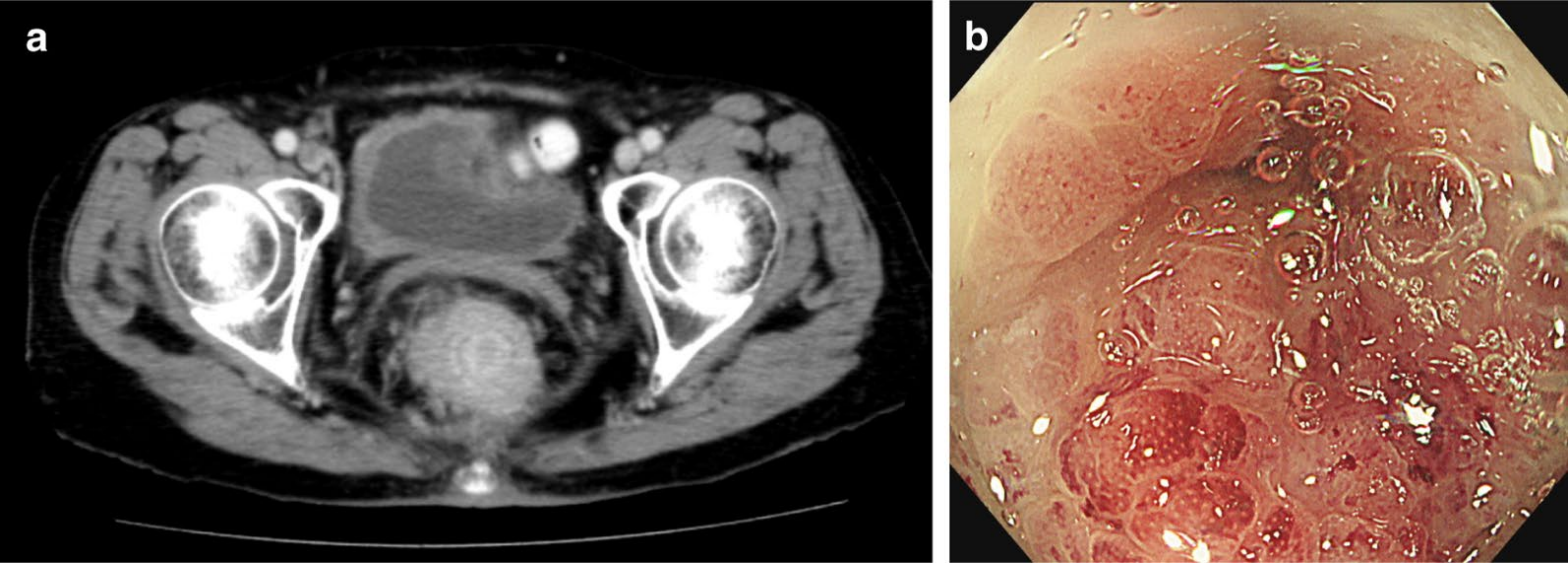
a Colonoscopy demonstrated an obstructive tumor in the rectum. b CT showed advanced rectal stenosis. CT, computed tomography
